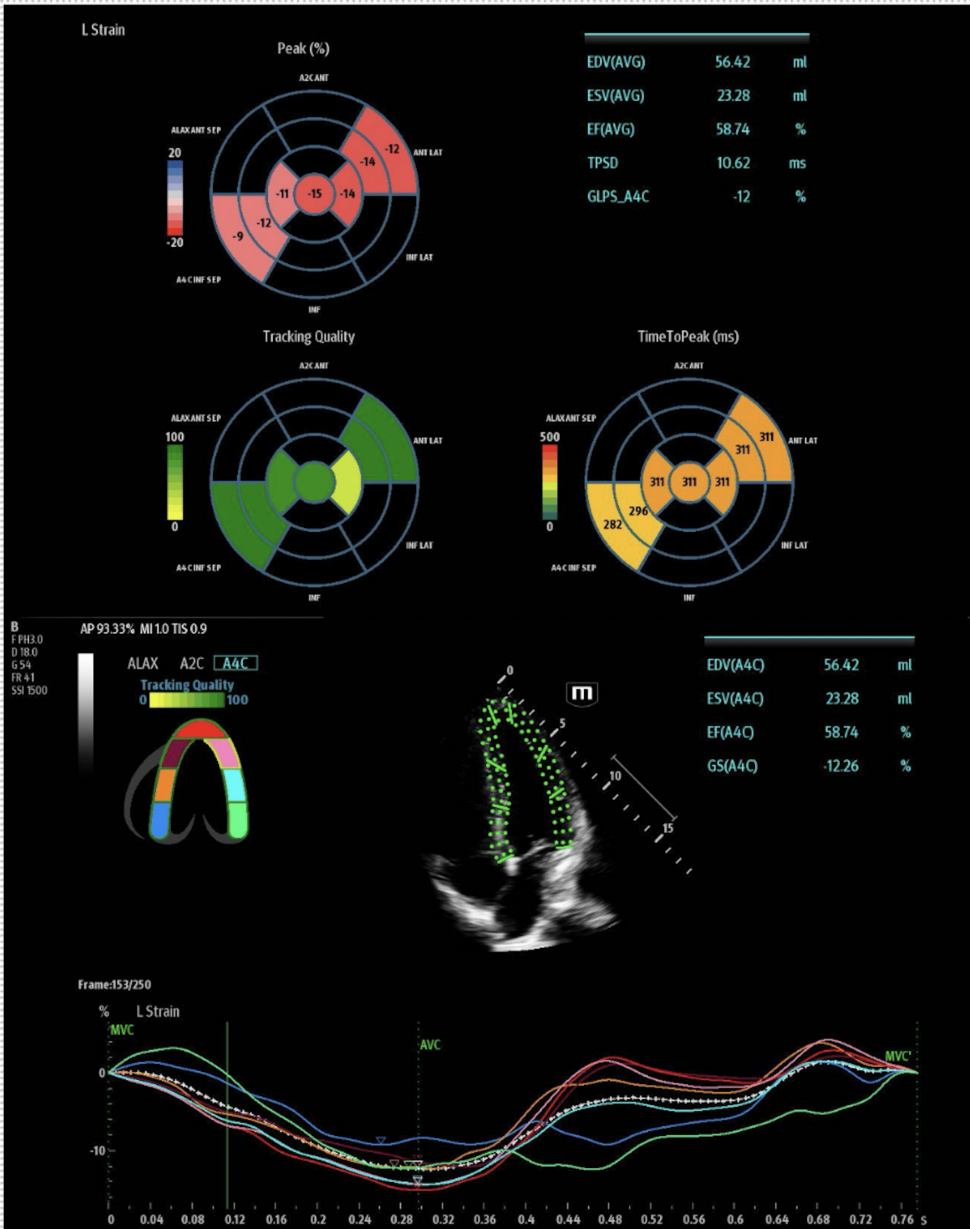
Limited speckle-tracking echocardiography on day two in the emergency department, showing a reduced global longitudinal strain and preserved ejection fraction
